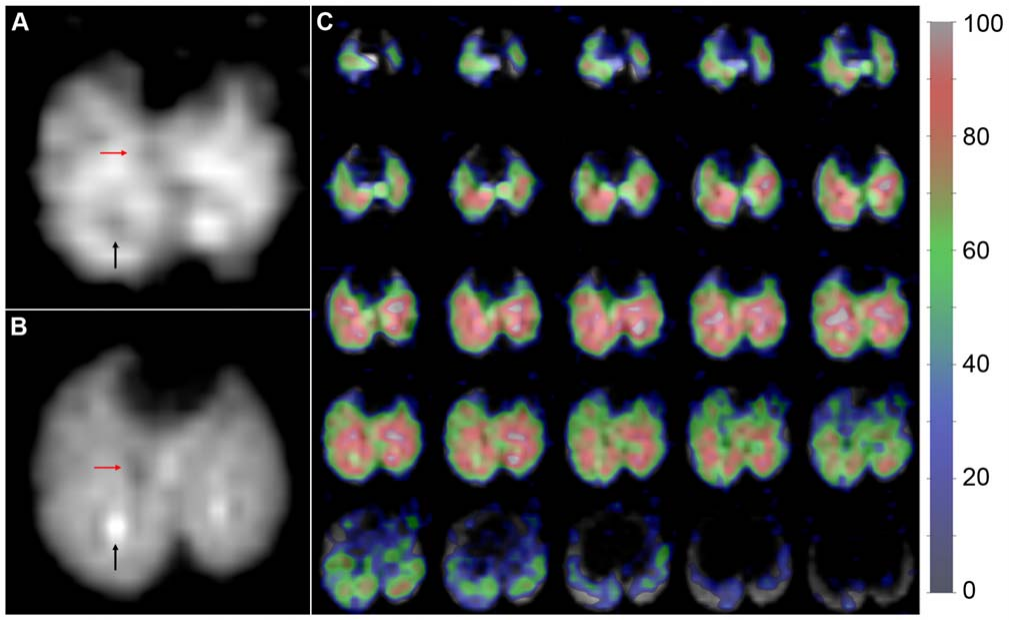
Gas-phase ^129^Xe MR imaging. A. Axial slice from an EC infusion image (EC flow ∼15 ml/min) acquired with a nominal isotropic resolution of 2×2×2 mm^3^. Black arrow indicates a signal void in the image corresponding to the location of a major airway. Red arrow indicates a hypointense region that likely corresponds to a large blood vessel. B. Axial slice from the corresponding ventilation image acquired with 1×1×2 mm^3^ nominal resolution. Black arrow indicates a large airway in the same location as the signal void in A (also marked by a black arrow). Red arrow points to a hypointense region corresponding to the red arrow in B. C. Complete 3D, gas-phase, EC infusion image (color) overlaid on the 3D ventilation image (grayscale). The signal intensity of the EC infusion image, in arbitrary units, is indicated in the legend.
